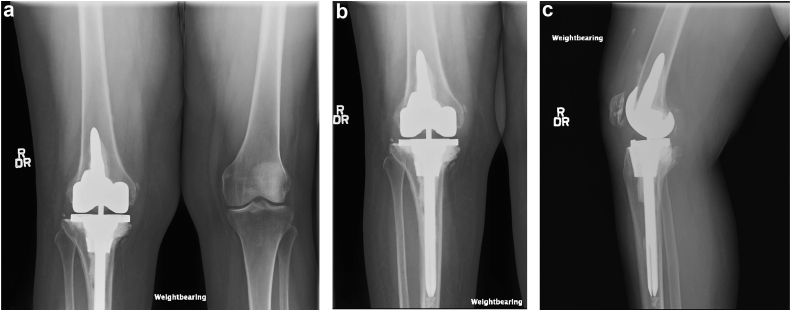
(a) Standing AP radiographs of bilateral knees at the patient’s 2-year postoperative visit show neutral mechanical alignment with well-positioned implants. (b) Standing AP radiographs of the right knee show a well-positioned, well-aligned constrained right TKA with a long-stemmed cemented tibial component and cone augmentation and short-stemmed femoral component. (c) Standing lateral radiograph of the right knee showing maintained alignment of the tibial component in the sagittal plane with cone augmentation and cementation providing adequate structural support to previous proximal tibial defect.
